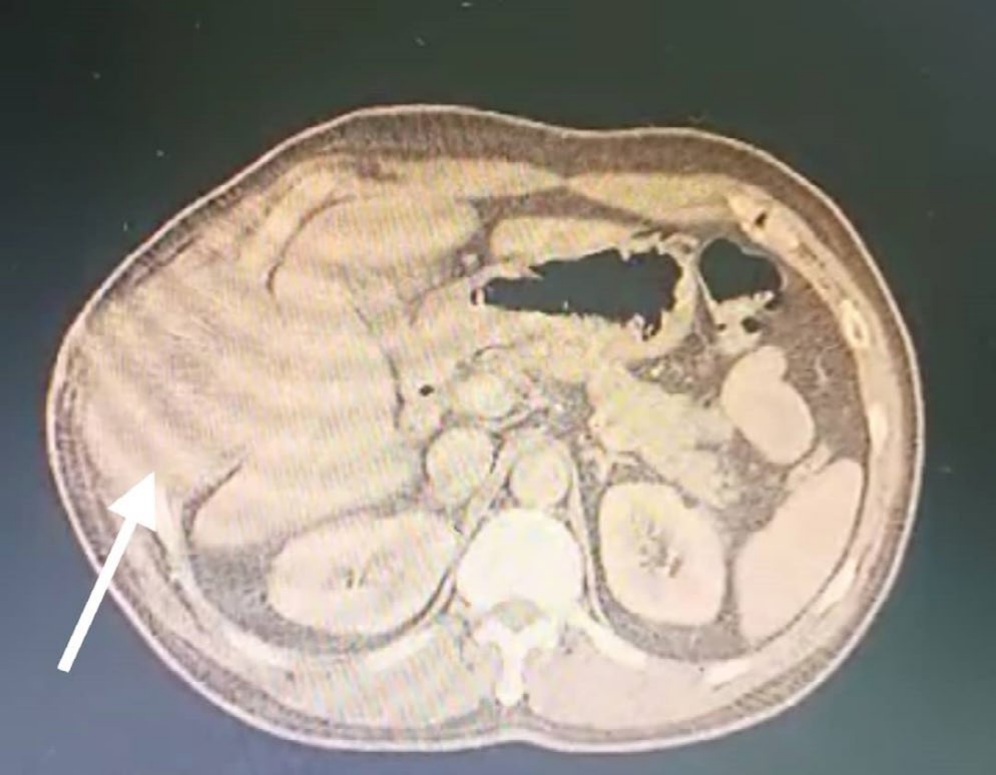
Axial CT of the lower thorax. Right posterolateral chest wall mass arising from the ribs (white arrow), with cortical irregularity/erosion and an associated soft‐tissue component. The lesion involves the right 9th–11th ribs in continuity.
